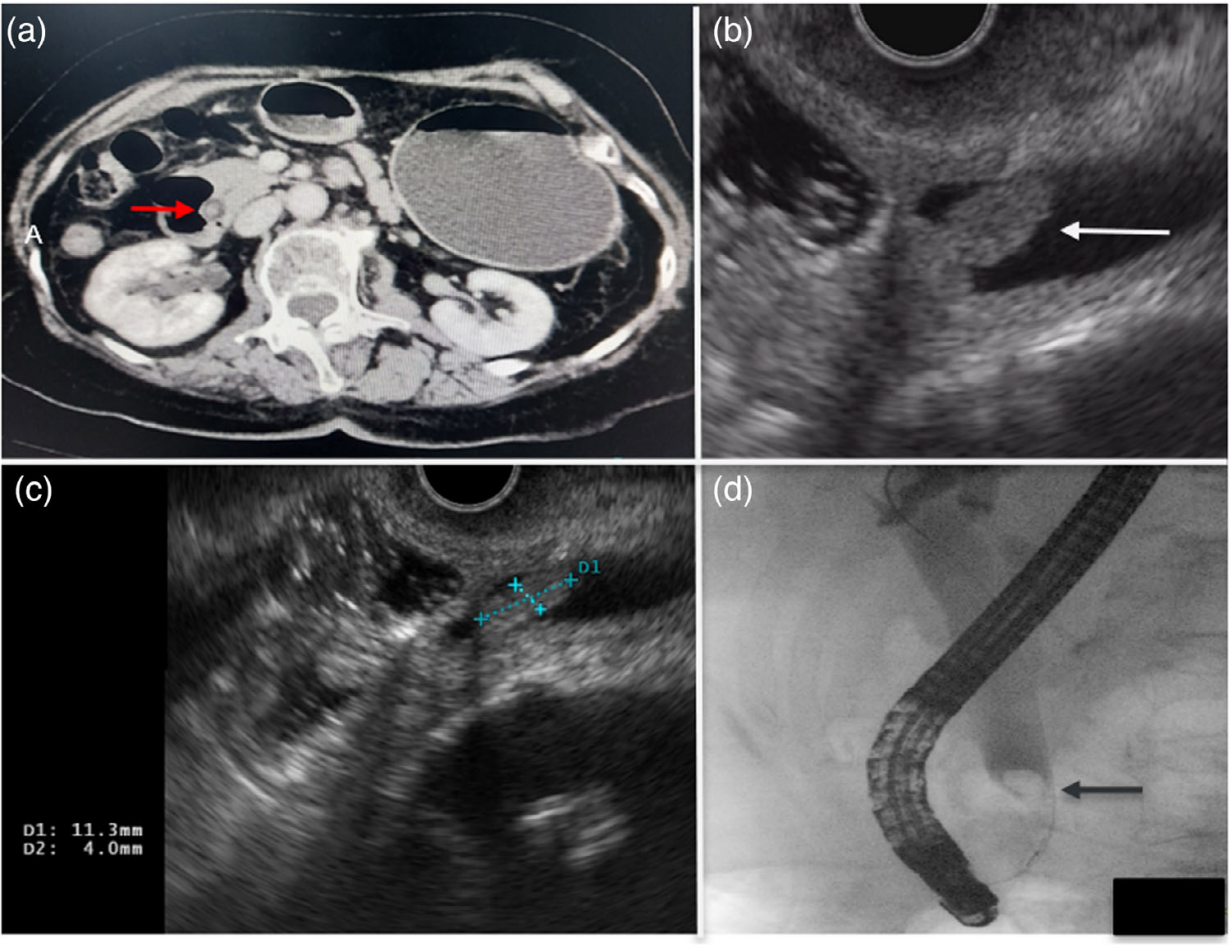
(a) Contrast‐enhanced abdominal computed tomography scan showing distal common bile duct polypoid lesion (red arrow). (b) Endoscopic ultrasound revealed a papillary mass with intraductal extension (white arrow). (c) Intraductal extension of the papillary mass measured 1.13 × 0.4 cm on endoscopic ultrasound. (d) Cholangiogram showed a filling defect within the papilla of Vater, extending proximally into the intraductal portion of the common bile duct.
